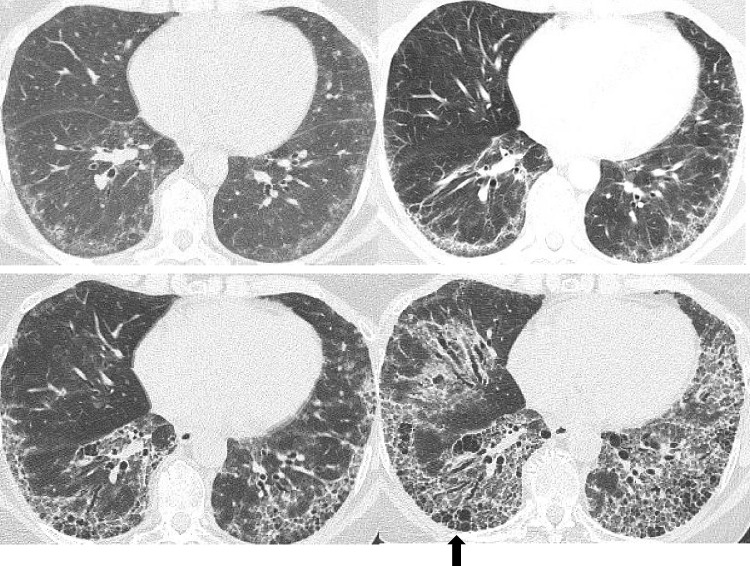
Serial chest HRCT scans of a 57-year-old female with SSc over the course of 8 years demonstrate the progression of interstitial fibrosis to such an extent that despite the initial HRCT (top left) demonstrating findings highly suggestive of NSIP, the most recent imaging (bottom right) shows advanced fibrotic ILD and honeycombing (black arrow) and looks more like UIP. HRCT, high-resolution computed tomography; NSIP, non-specific interstitial pneumonia; ILD, interstitial lung disease; UIP, usual interstitial pneumonia
